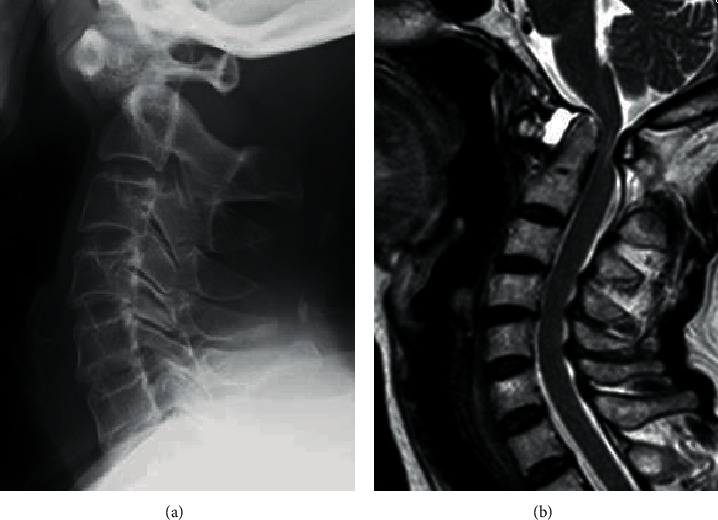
Side views of plain X-ray lateral standing images show atlantoaxial subluxation (a), and MRI confirms high compression of the spinal cord by the posterior arch of C1 (b).
